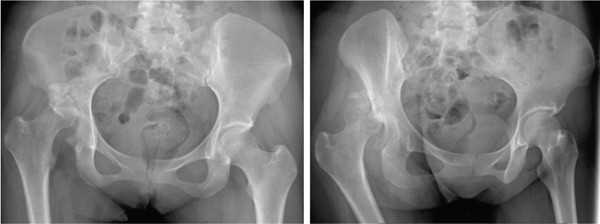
Case 4 preoperative radiographs.
